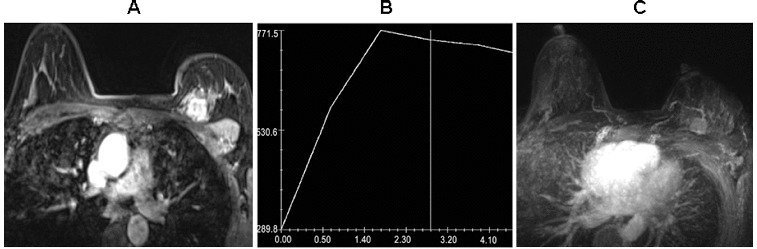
Invasive ductal carcinoma obtained from a 33-year-old woman. (A) Axial fat-suppressed contrast-enhanced axial image showing a 2.0 × 1.6 cm lobulated mass with heterogeneous enhancement in the upper-inner quadrant of the left breast. (B) Time–signal intensity curve of the mass shows a type III (washout) time curve. The *x*-axis shows the dynamic imaging beginning time in seconds, and the *y*-axis shows the intensity in arbitrary units. The mass was classified as a BI-RADS category IV lesion. (C) Maximum intensity projection reconstruction obtained from 3D T1-weighted subtraction image showing an adjacent vessel entering the mass.
